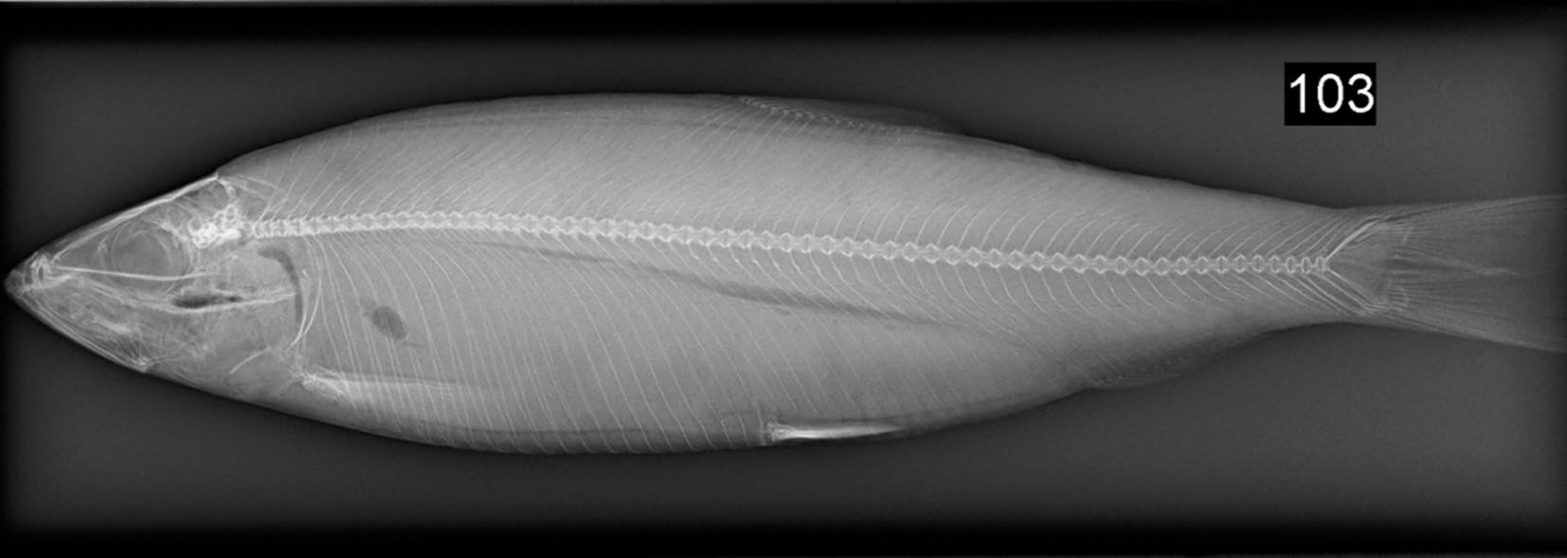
Radiograph from an Atlantic herring (*Clupea harengus*) used for visual inspections for internal trauma and deformities following trawling and pumping. No signs of spinal fracture or any other types of internal injuries (e.g., compressions, emphysemata and fluid accumulations) were found on any of the inspected individuals.
